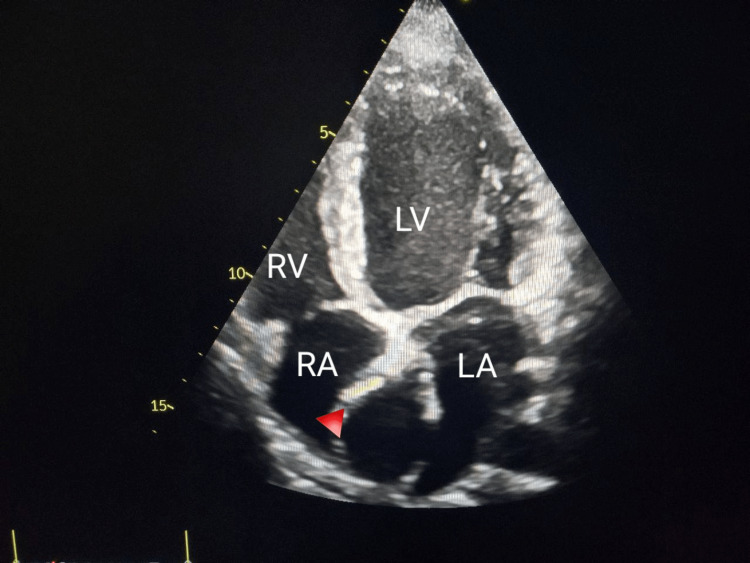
An echocardiogram apical four-chamber view showing mass compression in the right atrium (arrowhead). RA: right atrium; RV: right ventricle; LA: left atrium; LV: left ventricle
